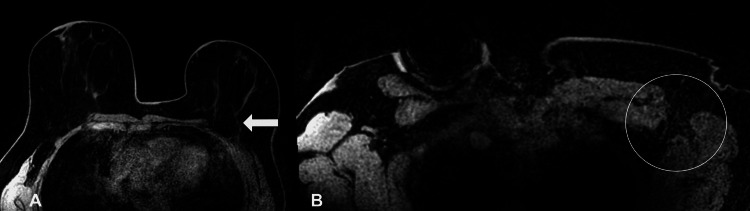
Post-treatment MRI of the breast and axilla. (A) Left breast MRI showing linear non-mass enhancement at the prior tumor site (arrowhead), consistent with treatment response and no residual mass. (B) Left axilla MRI showing marked reduction in lymphadenopathy (open circle), indicating favorable response to therapy.
